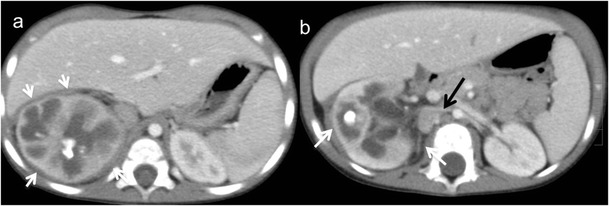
Diffuse XPN. a and b Contrast-enhanced nephrogenic phase transverse CT shows the massively enlarged affected kidney with inflammatory infiltration of perirenal fat (*white arrows*) and presence of adenopathy (*black arrow*)
